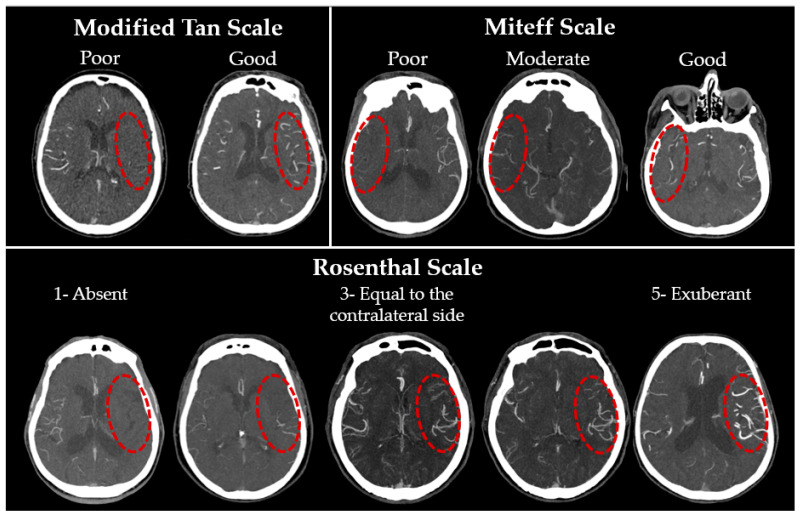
CT angiograms showing examples of collateral status grades for the modified Tan scale, the Miteff scale, and the Rosenthal scale. The areas indicated with a red dashed line indicate the side of the stroke.
